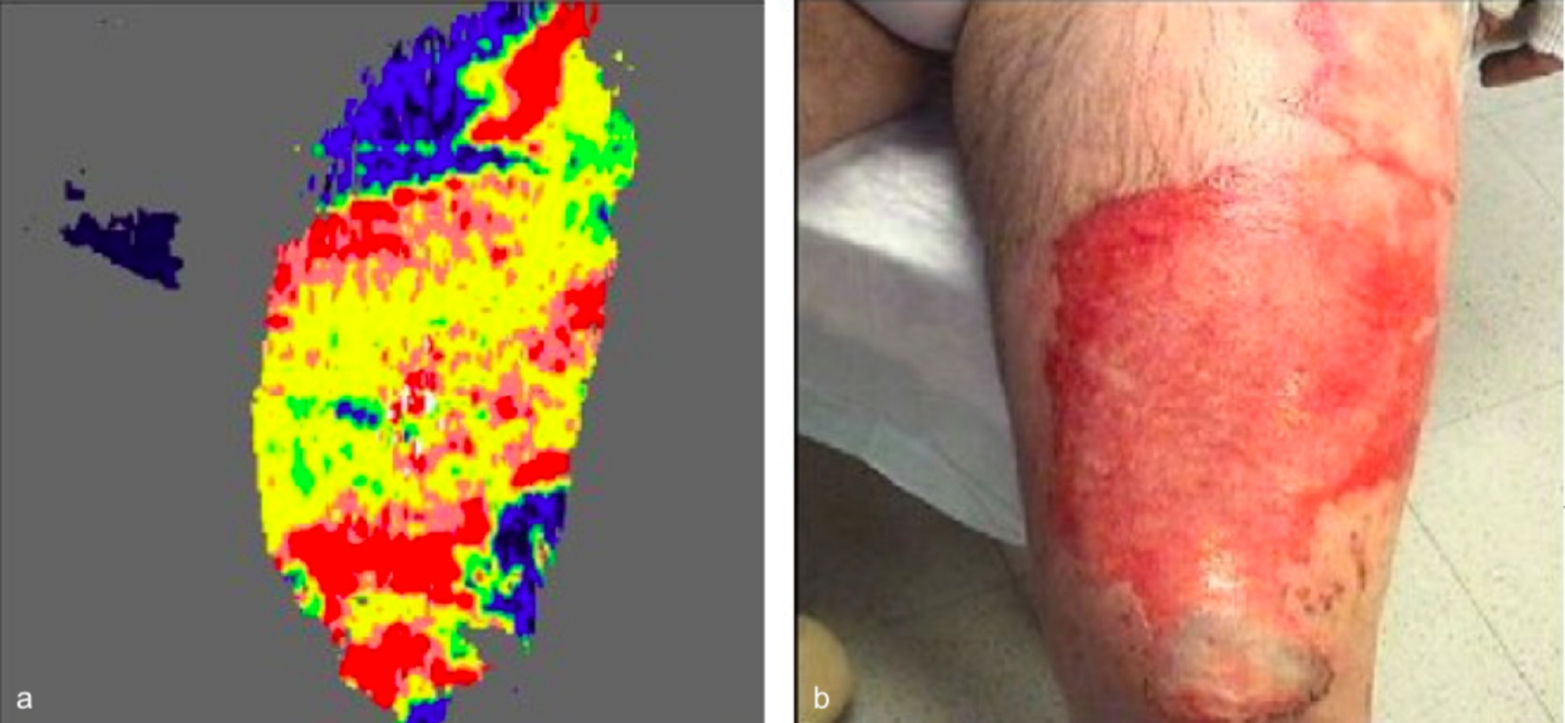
Laser Doppler imaging (LDI) performed 48 hours post-injury on anterior aspect of the left thigh and knee. LDI (a) and photo (b).
